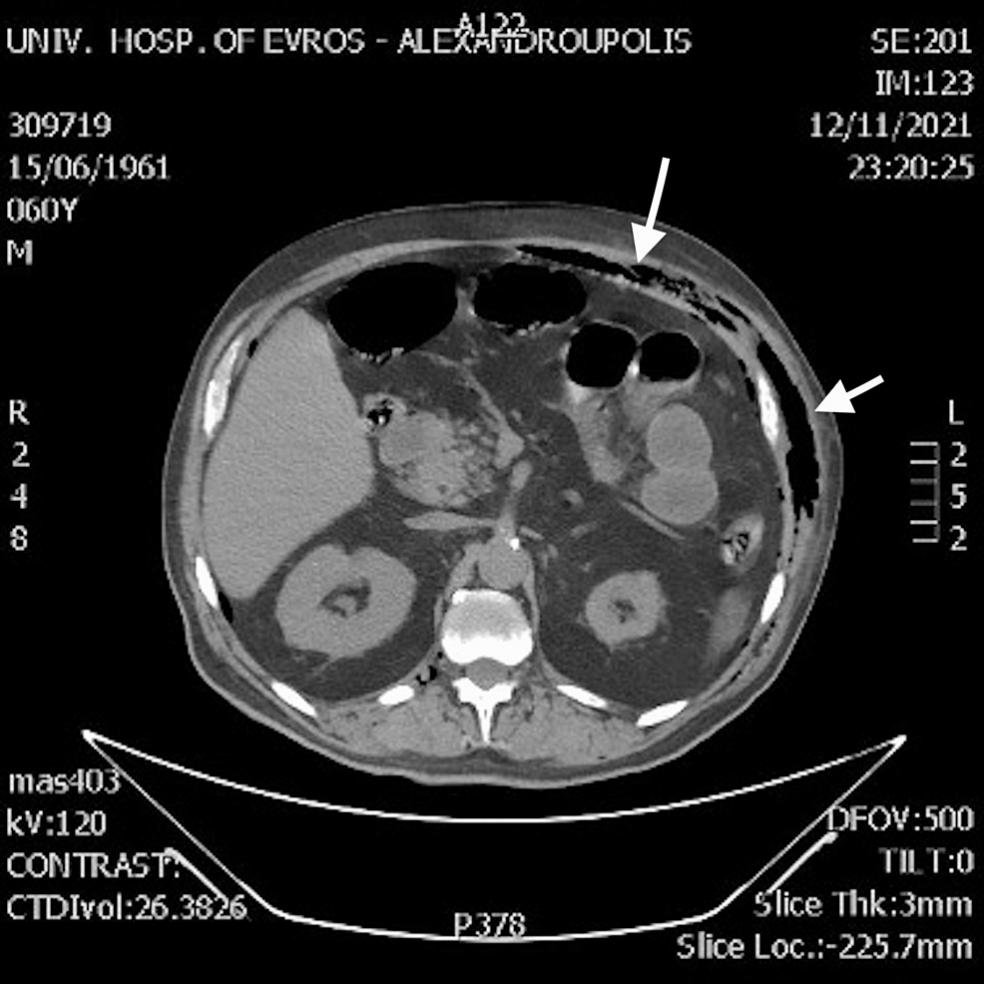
CT examination of the abdomen revealed free air in the abdominal wall (white arrows). CT: computed tomography.
